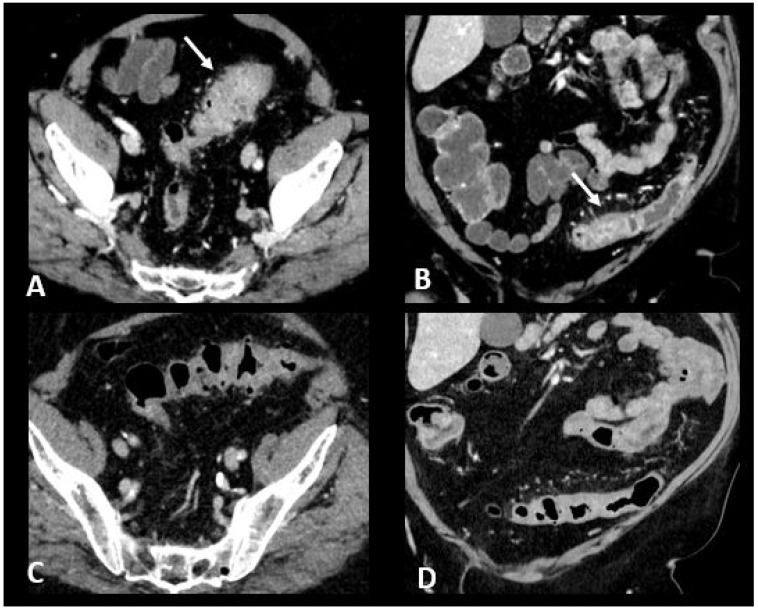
Immune-related colitis in a patient with a lung epidermoid carcinoma treated with nivolumab + ipilumimab. After 2 cycles of immunotherapy, the patient experienced grade 3 diarrhea. Transverse (A) and coronal (B) CT images show a segmental thickening of the sigmoid mimicking sigmoid diverticulitis (white arrows). The CT images in transverse (C) and coronal sections (D) performed after treatment cessation show a significant regression in the thickening of the sigmoid wall.
